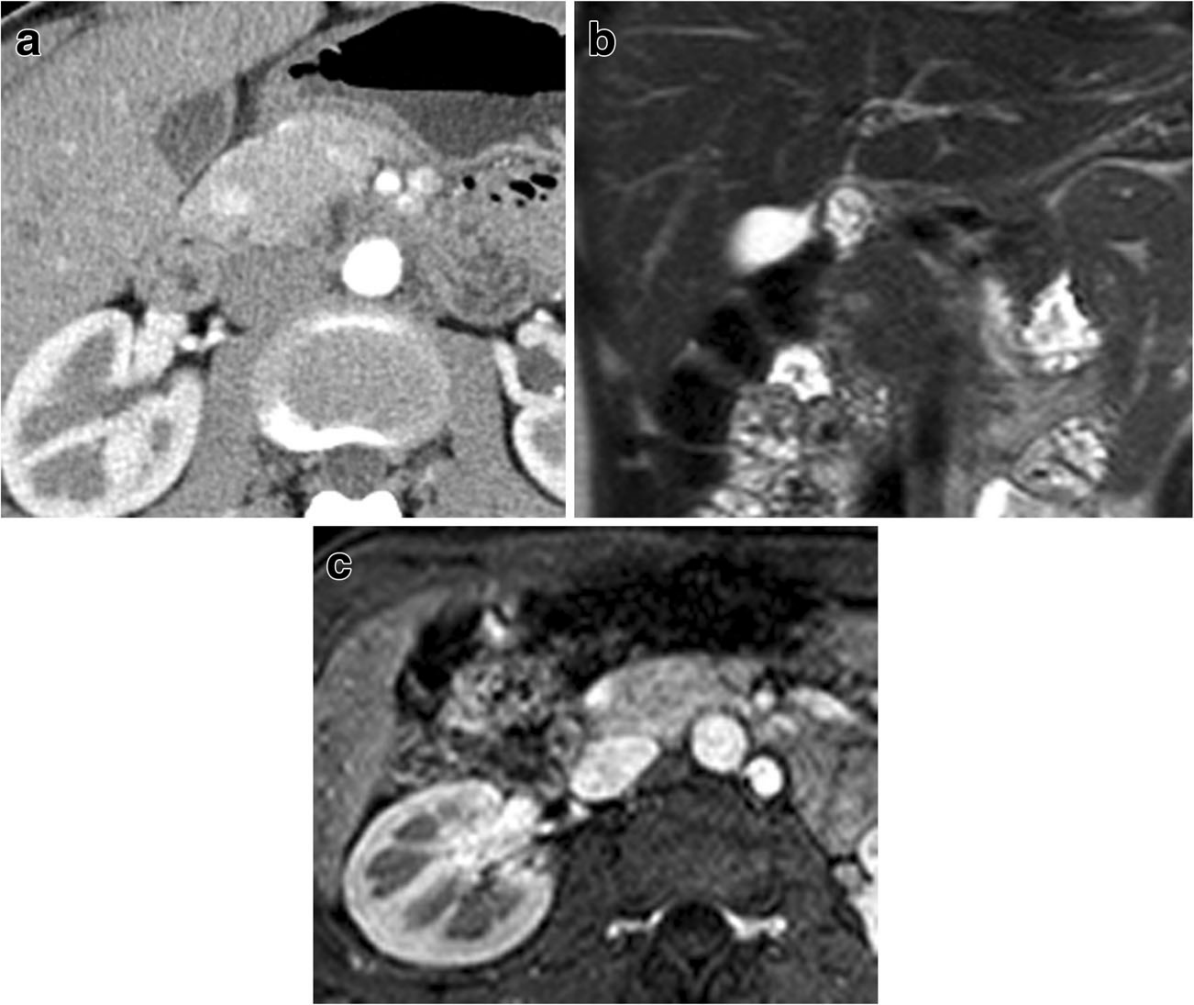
Insulinoma. CT: a small insulinoma appearing as a hypervascular hyperdense nodule (a) in the pancreatic head. MRI: the small insulinoma is well detectable as a hyperintense nodule (b) on T2-weighted coronal images and hypervascular (c) in the dynamic phase
